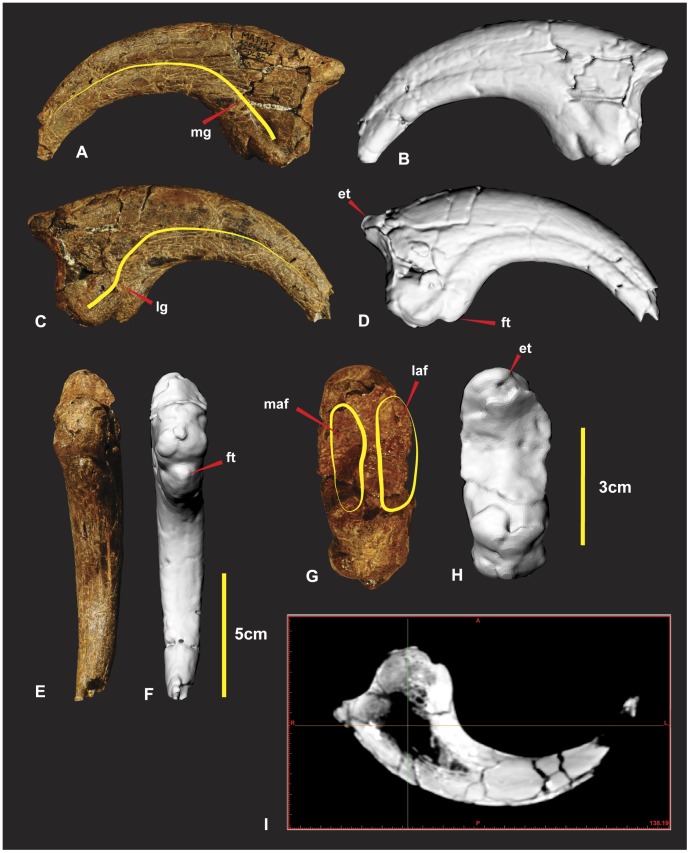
Right manual phalanx II-3. Right manual phalanx II-3 in medial (A & B), lateral (C & D), ventral (E & F), proximal (G & H), central proximo-distal CT image (I). *Abbreviations:* ex, extensor tubercle; ft, flexor tubercle; laf, lateral articular facet; maf, medial articular facet.
